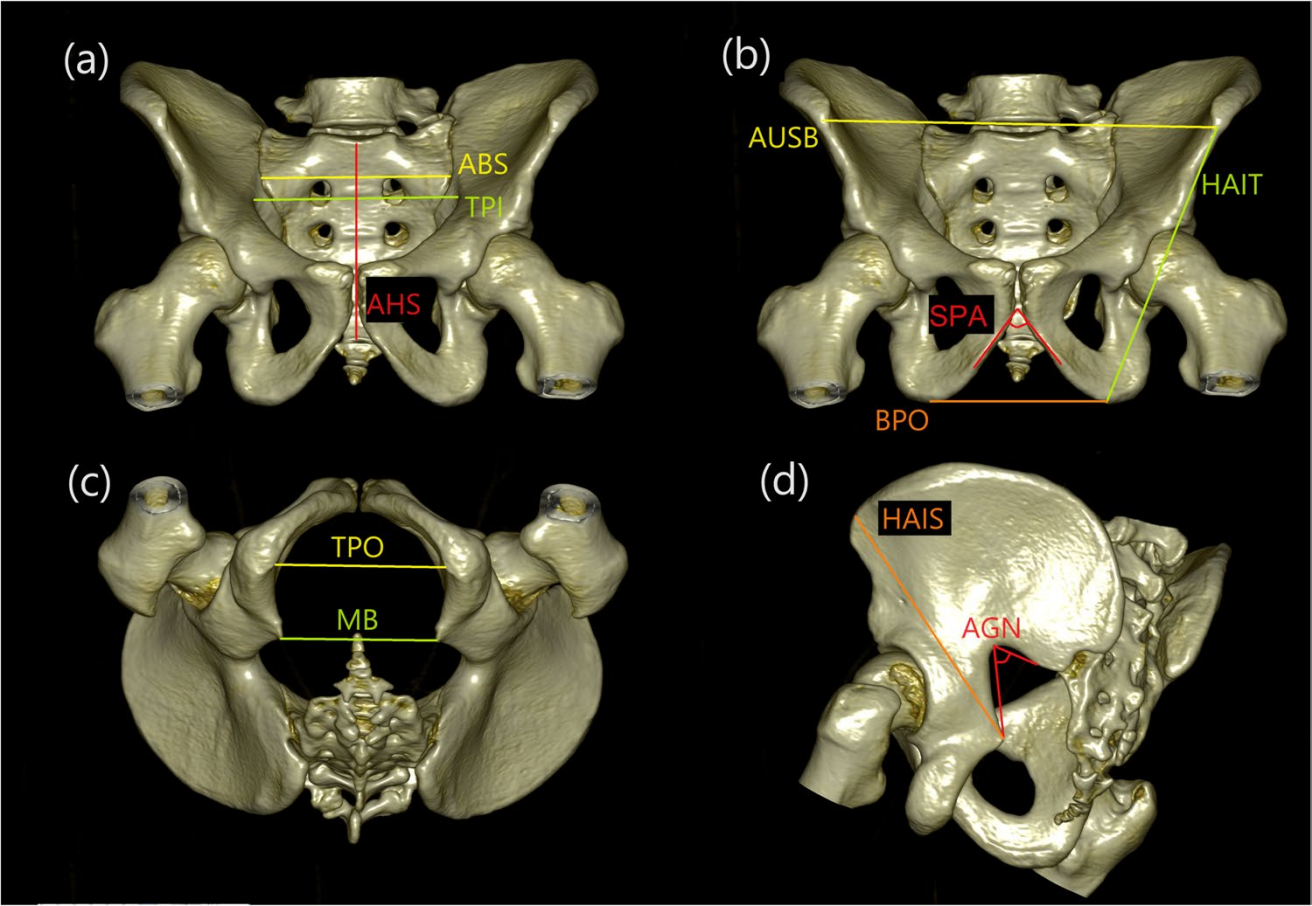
Three-dimensional computed tomography images showing pelvic measurements (see Table 2 for definition): a transverse pelvic inlet (TPI), anterior breadth of the sacrum (ABS), and anterior height of the sacrum (AHS); b subpubic angle (SPA), breadth of pelvic outlet (BPO), anterior upper spinal breadth of pelvis (AUSB), and height between anterior superior iliac spine and anteroinferior margin of ischial tuberosity (HAIT); c transverse pelvic outlet (TPO) and midpelvic breadth (MB); and d angle of greater sciatic notch (AGN) and height between anterior superior iliac spine and ischial spine (HAIS)
